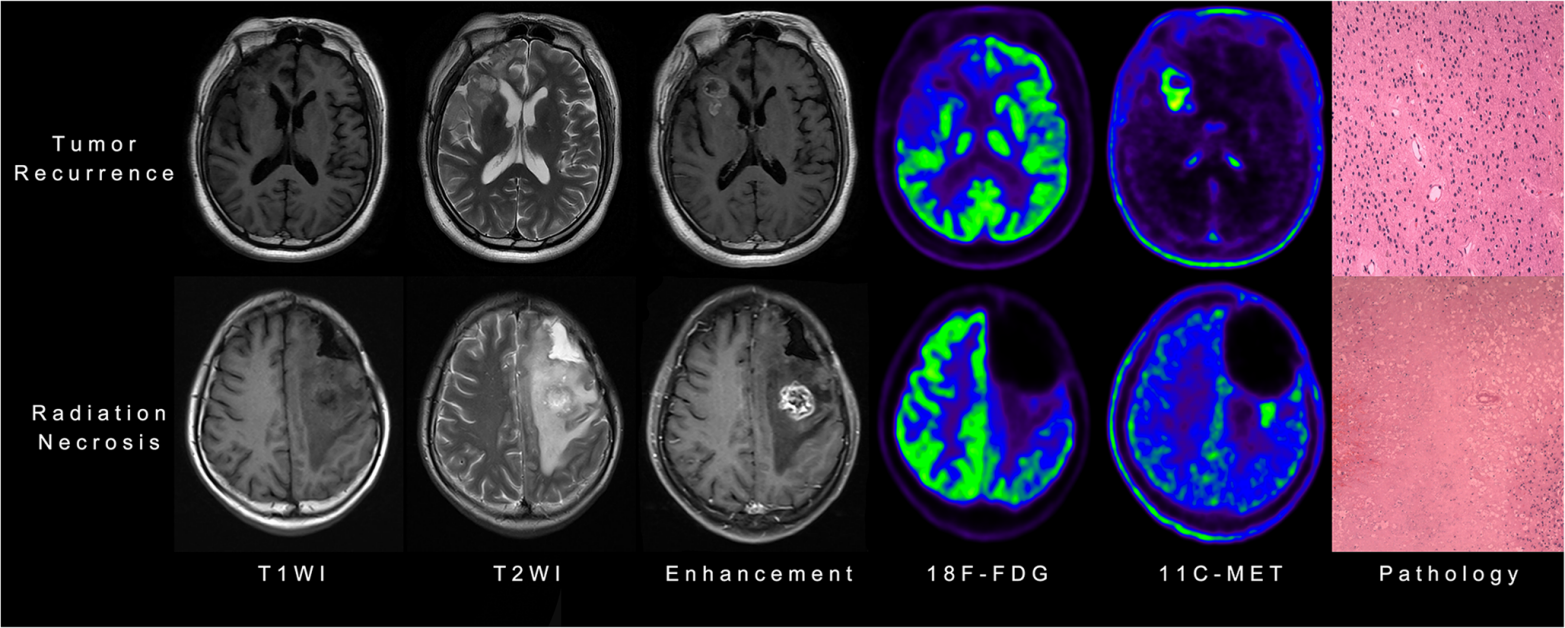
Representative MRI and PET images showing features of tumor recurrence and radiation necrosis, including images from T1-weighted (T1WI), T2-weighted (T2WI), and contrast-enhanced T1W1 MRI, ^18^F-fluorodeoxyglucose (^18^F-FDG) and ^11^C-methionine (^11^C-MET) PET, and pathological analyses
